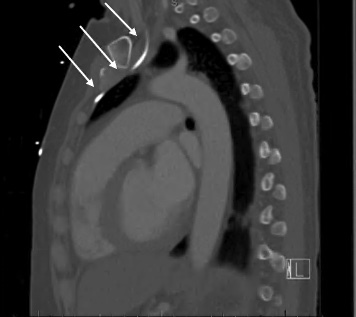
Saggital slice of chest computed tomography demonstrates the central venous catheter within the internal mammary vein, posterior to the sternum, coursing anterior to the great vessels and pleural space (white arrows). Volume 7, no. 1: February 2023 53 Clinical Practice and Cases in Emergency Medicine
